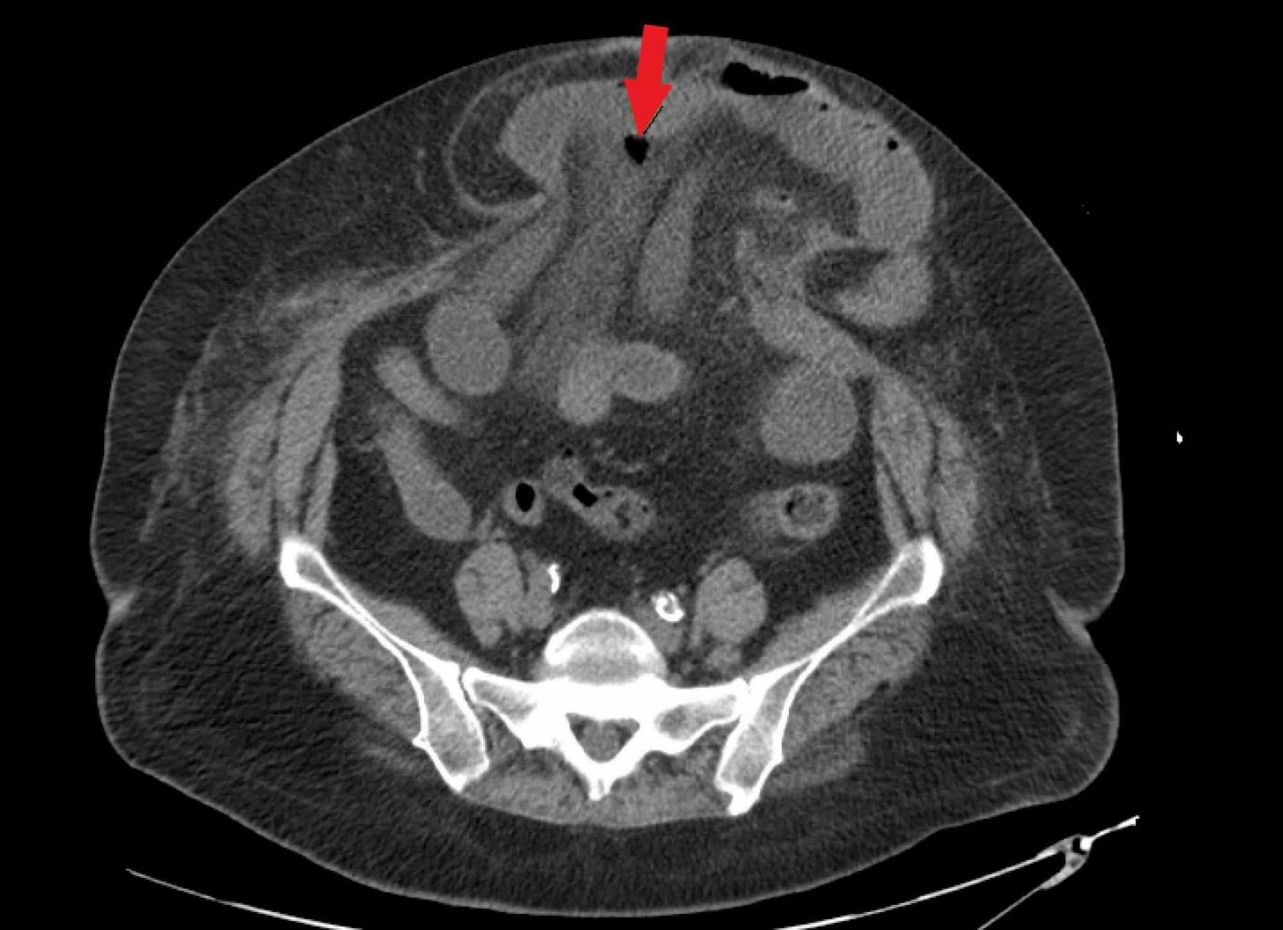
Repeat CT scan showing a small amount of free air (see arrow) adjacent to loops of small bowel within the ventral hernia and associated inflammatory changes
